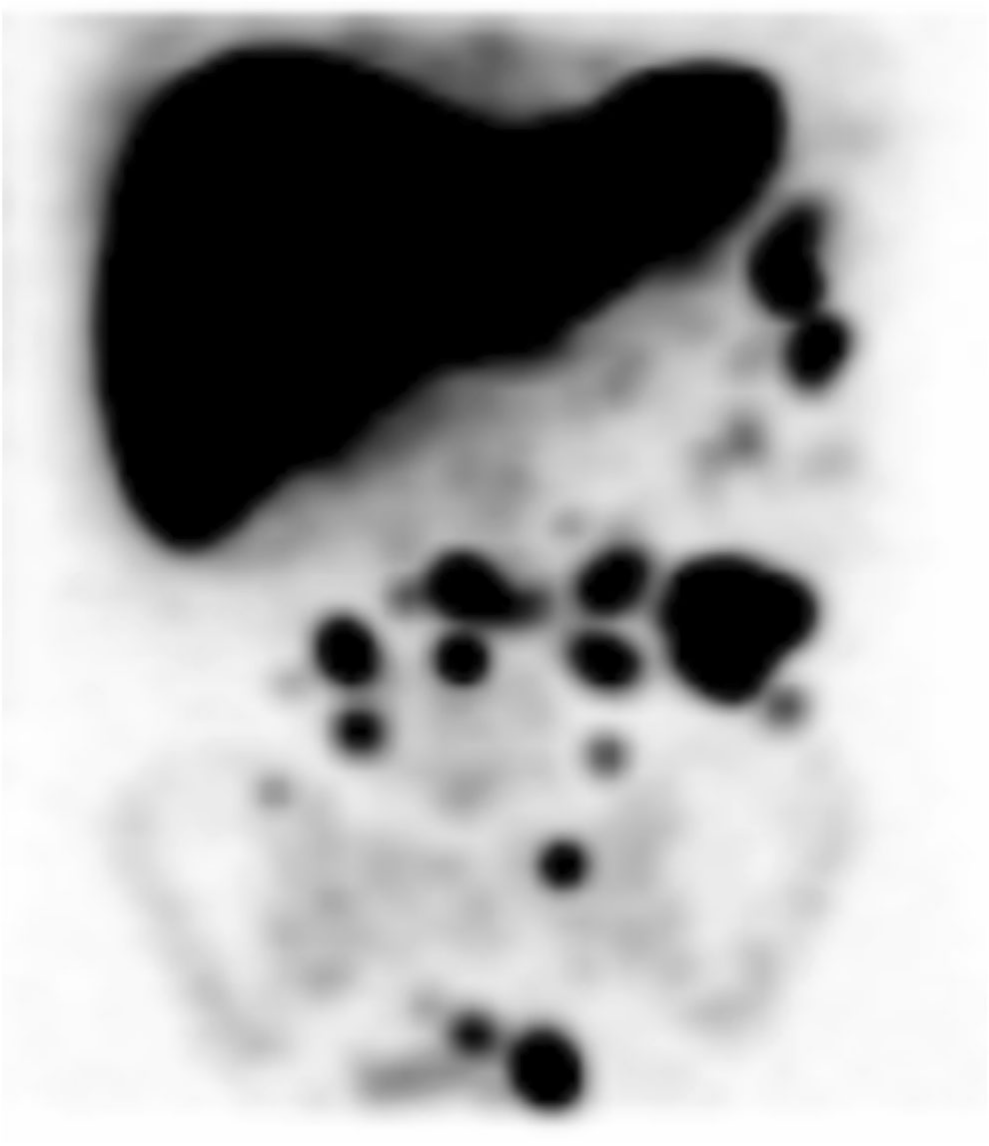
Maximum intensity projection of SPECT giving an overview of the extent of disseminated splenosis within the abdomen and pelvis
